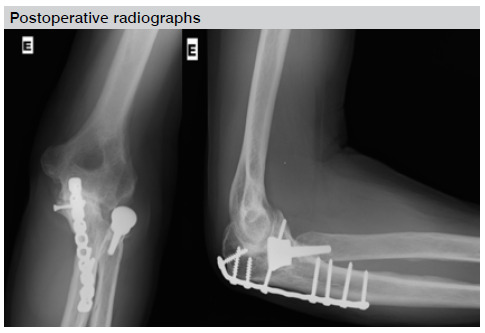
Posterolateral rotatory instability of the elbow as a complication. Postoperative radiographs
